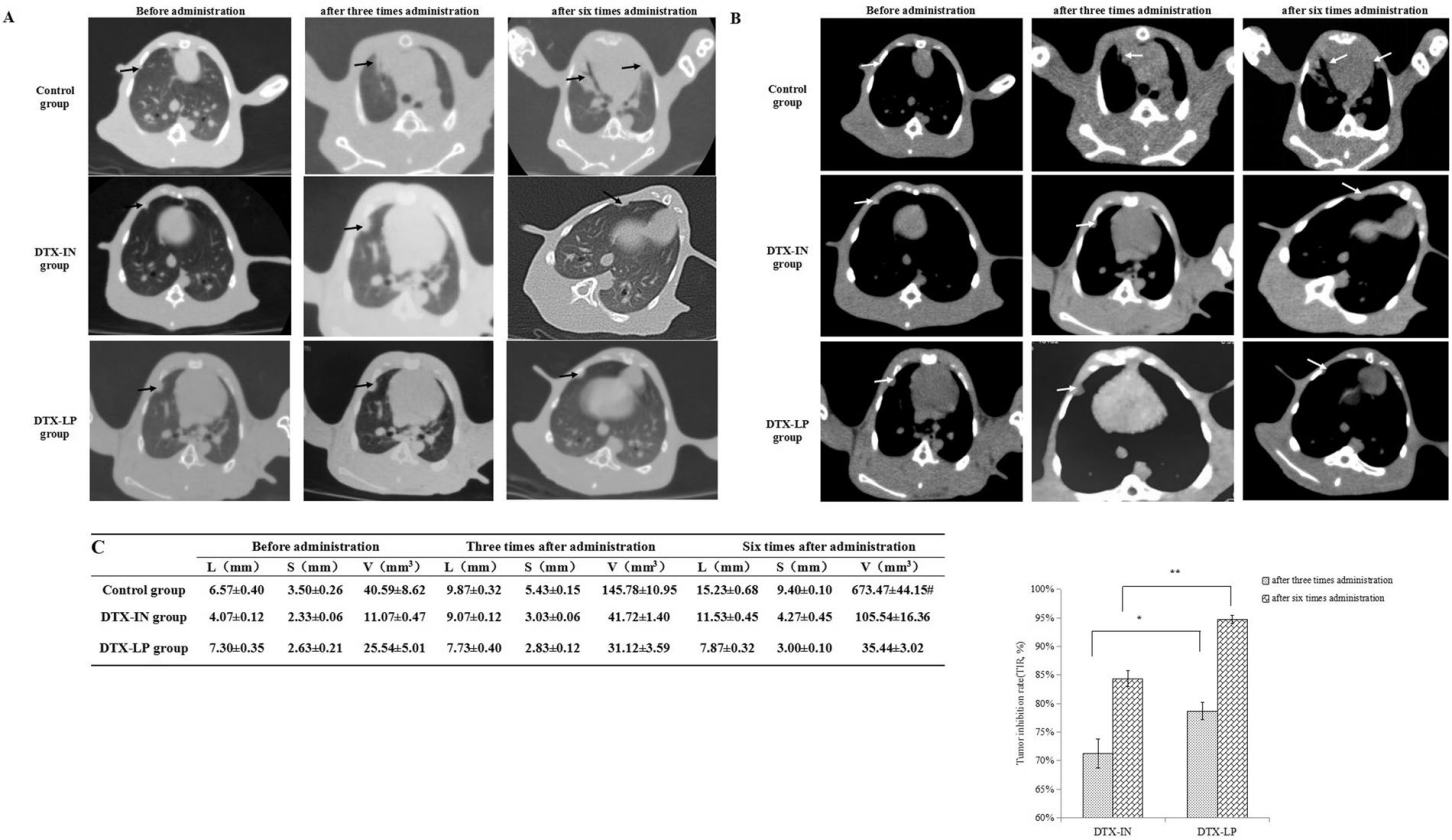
CT imaging. (A) Pulmonary window (black arrow means the single and localized intrapulmonary tumor), (B) Mediastinal window (white arrow means the single and localized intrapulmonary tumor); (C) the volumes of tumors (mm^3^) calculated from CT imaging measured by experienced technician (n = 3, ^#^the tumor had been spread to the chest), (D) TIR (%) calculated by formula previously described (t-test, *p* ≤ 0.05) p* < 0.05, *p* < 0.01.
